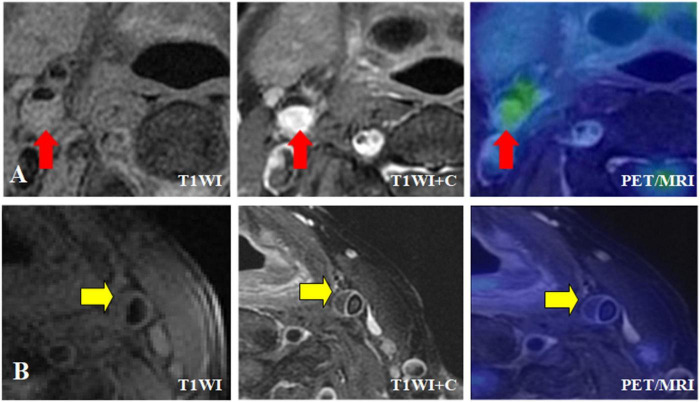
Different MRI types of carotid plaque integrated ^18^F-FDG PET/MR images. (A) Symptomatic patient with severe stenosis of right internal carotid artery lumen with plaque formation on T1WI, and the plaque was isointense. T1WI enhancement showed significant enhancement of right internal carotid artery plaque, and transverse fusion PET/MR showed high uptake of ^18^F-FDG, SUVmax = 3.5, TBR = 2.5. (B) Asymptomatic patient with moderate stenosis of left internal carotid artery with plaque formation, plaque showed equal signal, HR-MRI transverse axial T1WI showed obvious continuous band enhancement of left internal carotid artery plaque surface, no enhancement in plaque, transverse fusion PET/MR showed no uptake of plaque ^18^F-FDG, SUVmax = 2.05, TBR = 1.25.
